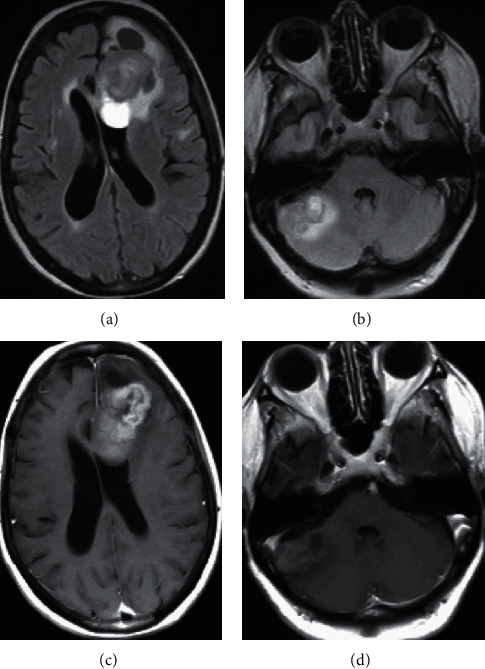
MRI showing the same lesions seen in the CT scan, suggestive of metastases. (a), (b) T2-FLAIR; (c), (d) contrast-enhanced T1-weighted.
